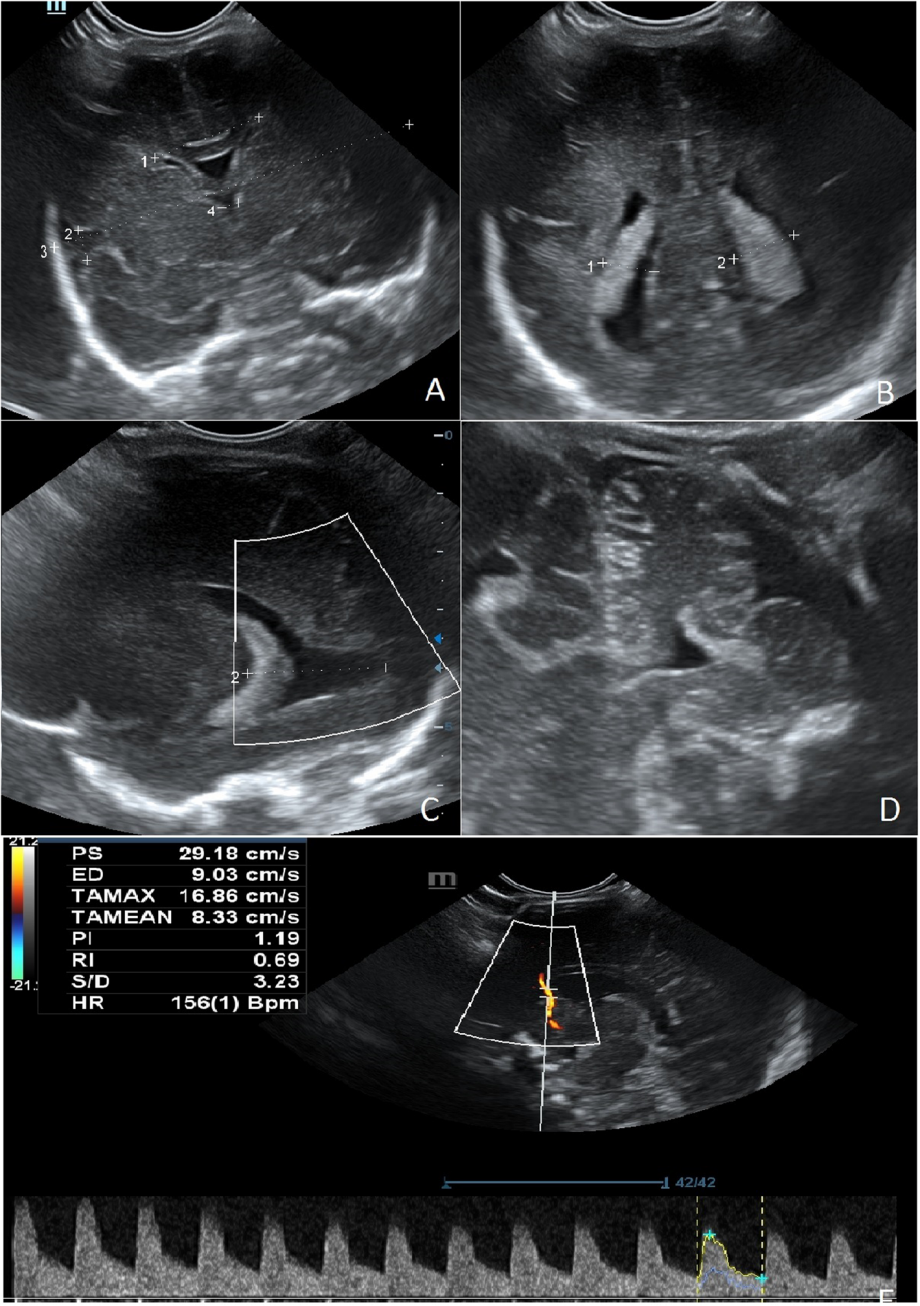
Cranial sonography at the age of 30 weeks corrected age. A Coronal scan showing normal frontal horns, cavum sepum pellucidi, third ventricle. B Choroid plexus in lateral ventricles in coronal scan. C Scan of normal occipital horn. D Scan of normal cerebellum visualized by mastoid window, E Normal Doppler parameters of blood flow in the arteria cerebri anterior. (written consent to publish was obtained from the patient’s parents)
